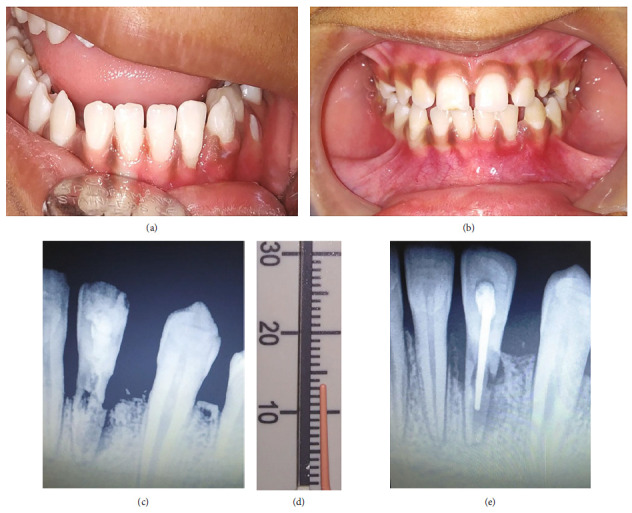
Case I: follow-up. (a) Frontal view after removal of splint at 2 weeks with adequate healing. (b) Frontal view showing slight inflammatory redness around tooth #32 after 3.5 years. (c) Radiograph showing root resorption till middle one-third of root at 3.5 years. (d) No. 80 Gutta Percha adjusted to 13 mm according to the suspected length of periodontal communication. (e) 20° angulated radiograph showing severe communicating internal and external root-resorption at 3.5 years.
